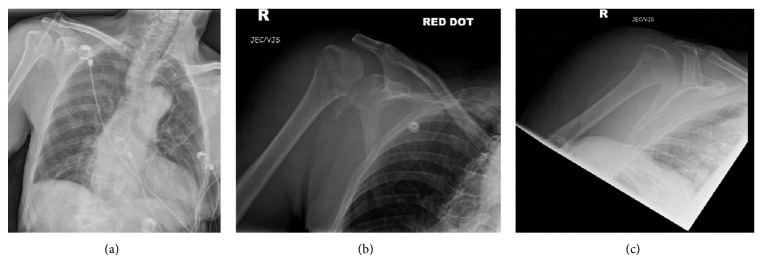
AP chest (a) and right shoulder AP (b) and Y-view (c) radiographs in A&E.
